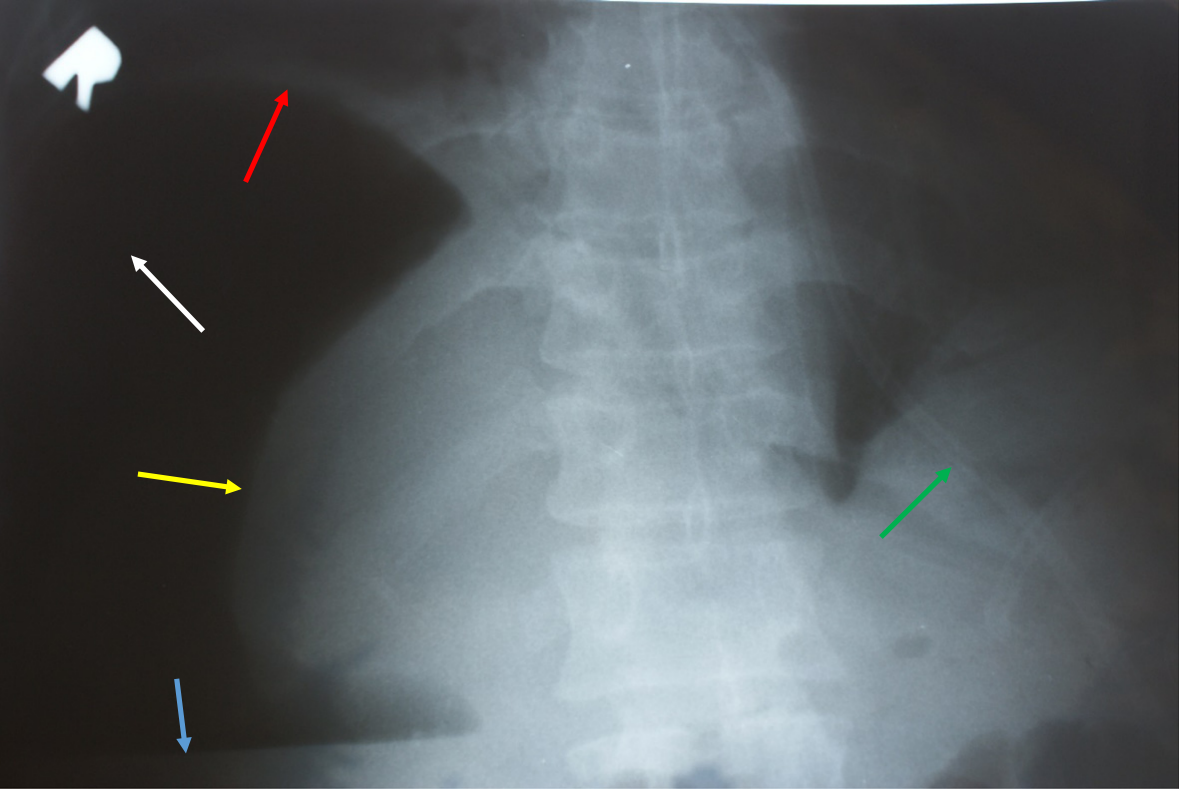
Erect plain abdominal X-ray on admission confirming tension pneumoperitoneum. Large amount of free gas under the right dome of the diaphragm, enveloping the liver (*white arrow*). The liver is diminished in size and has shifted downwards and medially, resembling a collapsed lung in pneumothorax: “the saddlebag sign” (*yellow arrow*). Liquid level in the free peritoneal cavity (*blue arrow*). High position of the right dome of the diaphragm (*red arrow*). Nasogastric tube (*green arrow*)
